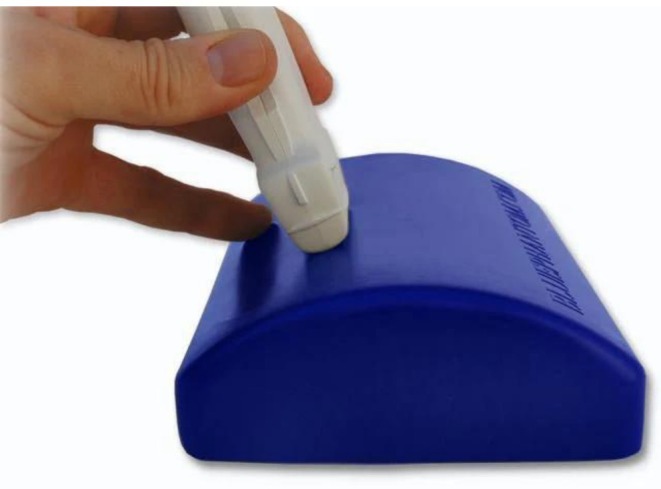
The regional anesthesia ultrasound training block.
